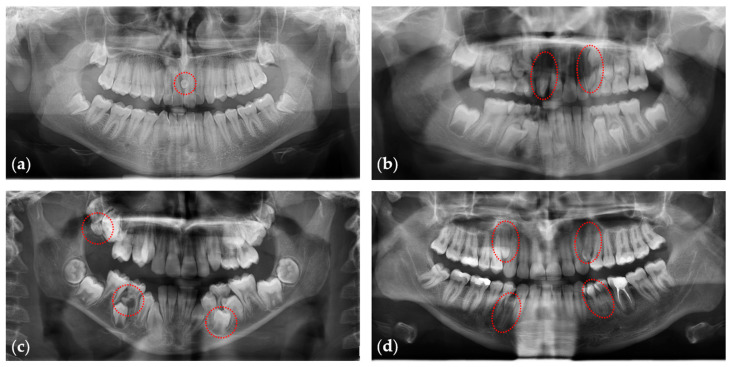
Panoramic radiographs showing individuals with (a) one, (b) two, (c) three, or (d) four supernumerary teeth, indicated by dashed red circles.
